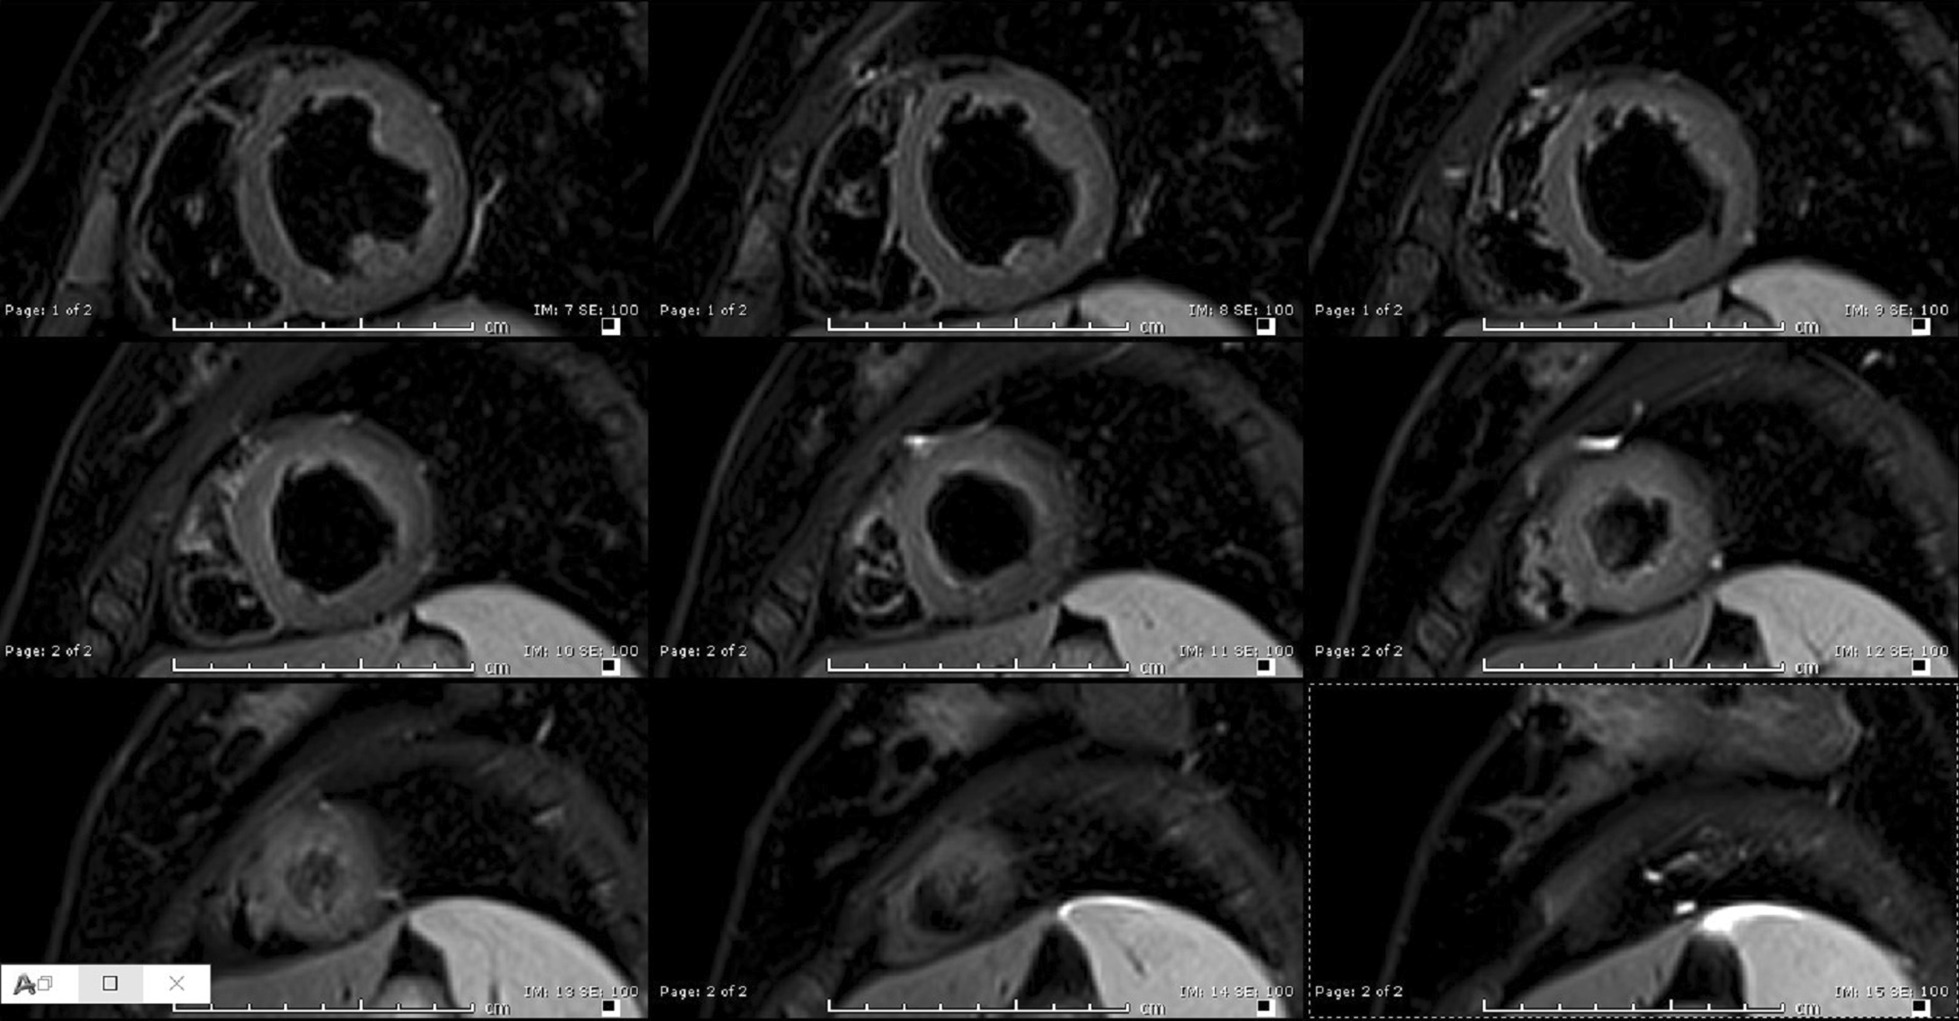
Case 1. T2-weighted short tau inversion recovery (STIR) imaging of the left ventricular (LV) short axis from base to apex. Diffuse increase in subendocardial signal intensity suggestive of subendocardial edema
